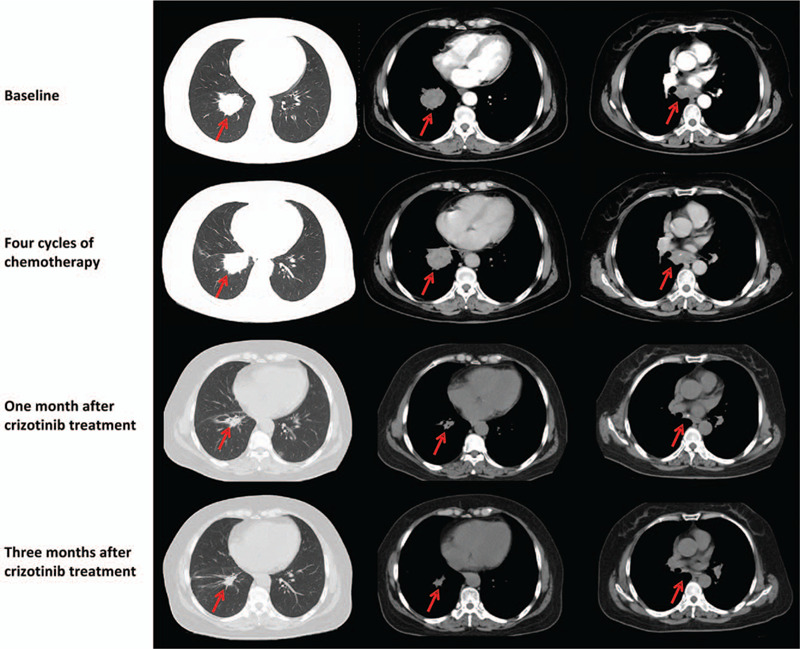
Computed tomography (CT) scans of patient before and after crizotinib treatment.
